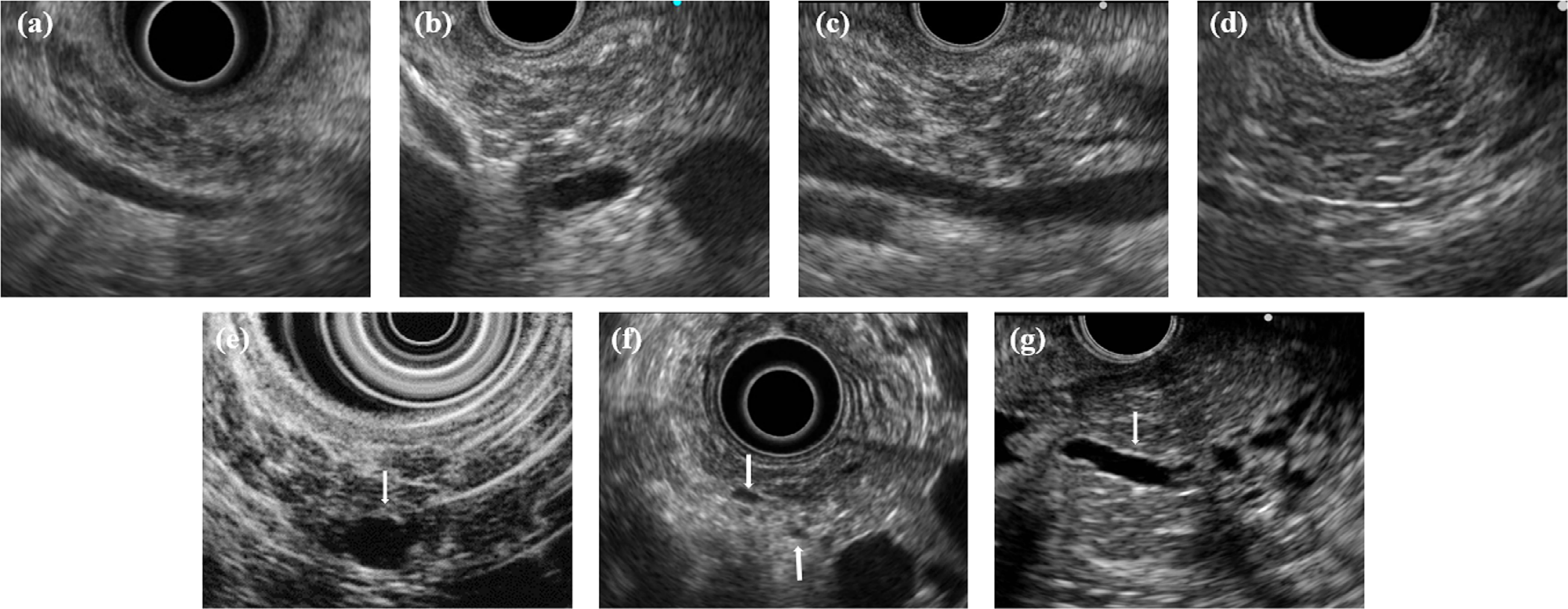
Endoscopic ultrasound findings of Rosemont classification for chronic pancreatitis: (a) Lobularity with honeycombing. (b) Lobularity without honeycombing. (c) Hyperechoic foci without shadowing. (d) Strands. (e) Cysts. (f) Dilated side branches. (g) Hyperechoic main pancreatic duct margin
